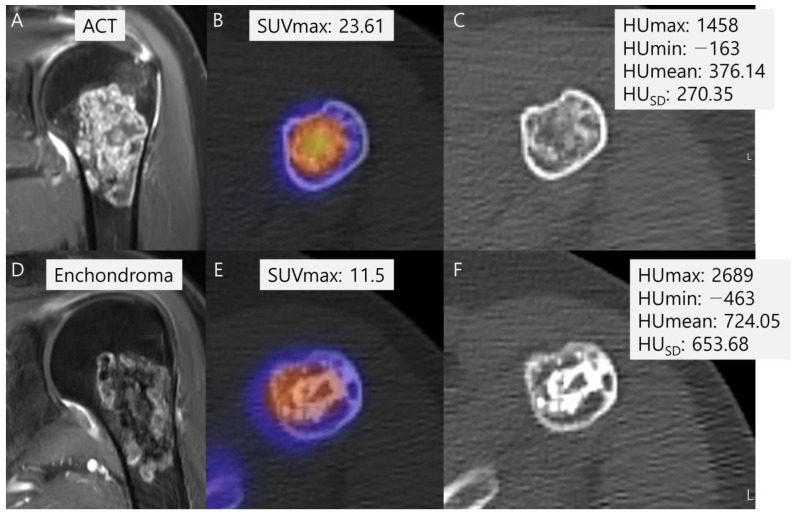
Comparison of SUVmax and radiodensity in HU measurements between ACT (A–C) and enchondroma (D–F). (A) A coronal T1-weighted fat-suppressed enhanced MRI shows a lobulated mass with septal enhancement with pathology of ACT. (B) A fused SPECT/CT image shows the radioactive uptake with SUVmax of this lesion which was calculated to be 23.61. (C) The radiodensity in HU measurement of this lesion was found to be 376.14 ± 270.35 [−163 to 1458]. (D) A coronal T1-weighted fat-suppressed enhanced MRI shows a lobulated mass with faint septal enhancement with pathology of enchondroma. (E) A fused SPECT/CT image shows the radioactive uptake with SUVmax of this lesion which was calculated to be 11.5. (F) The radiodensity in HU measurement of this lesion was found to be 724.05 ± 653.68 [−463 to 2689].
